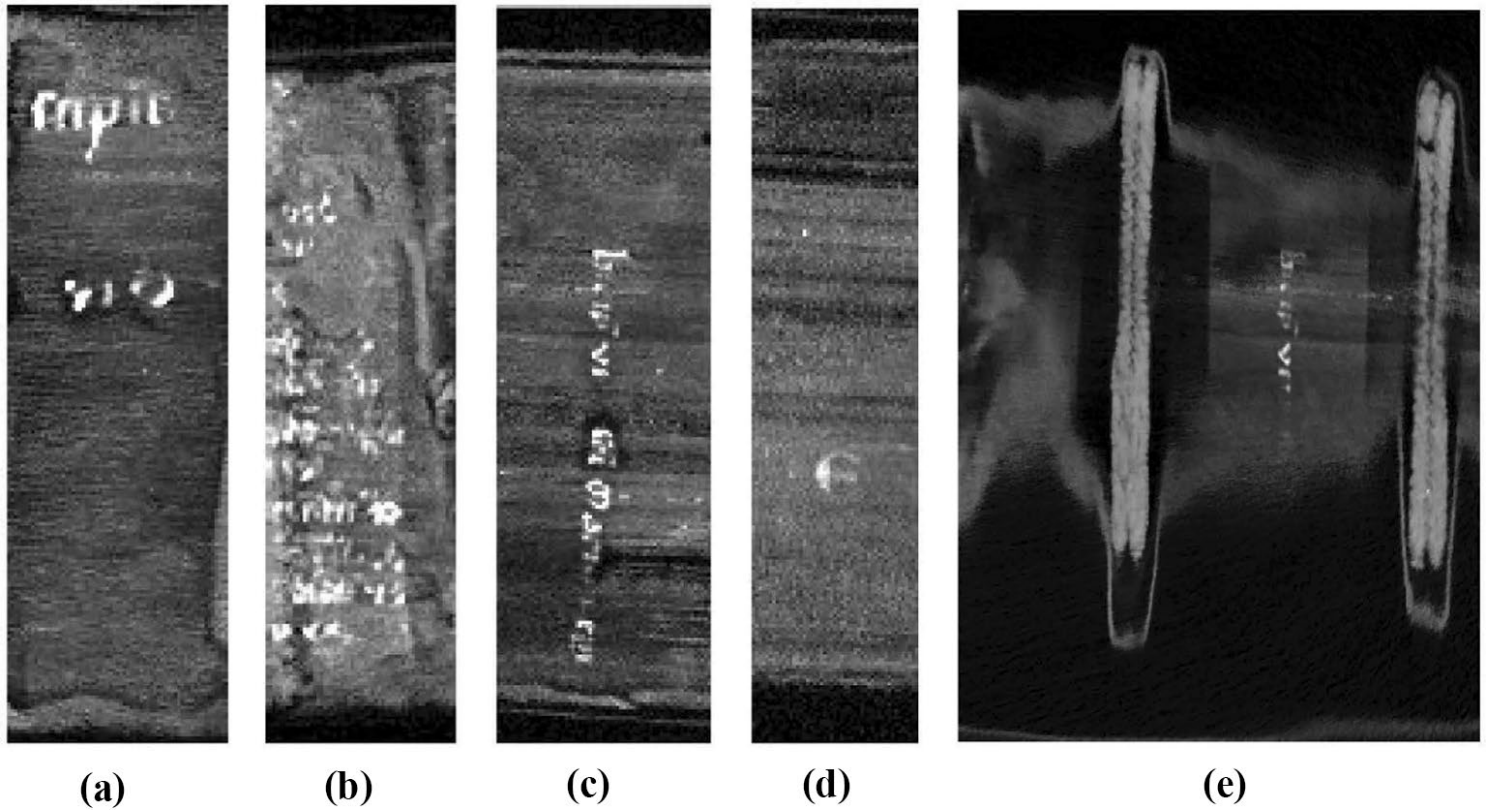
a-d LOGISMOS-flattened CT images of parchment with visible writing; e a single slice of the CT volume centered on the text that can be seen in the original CT volume prior to LOGISMOS-flattening. Note that while it is possible to determine presence of text in the original CT volume, LOGISMOS-flattening facilitates the visualization and reading of a much larger portion as shown in c
